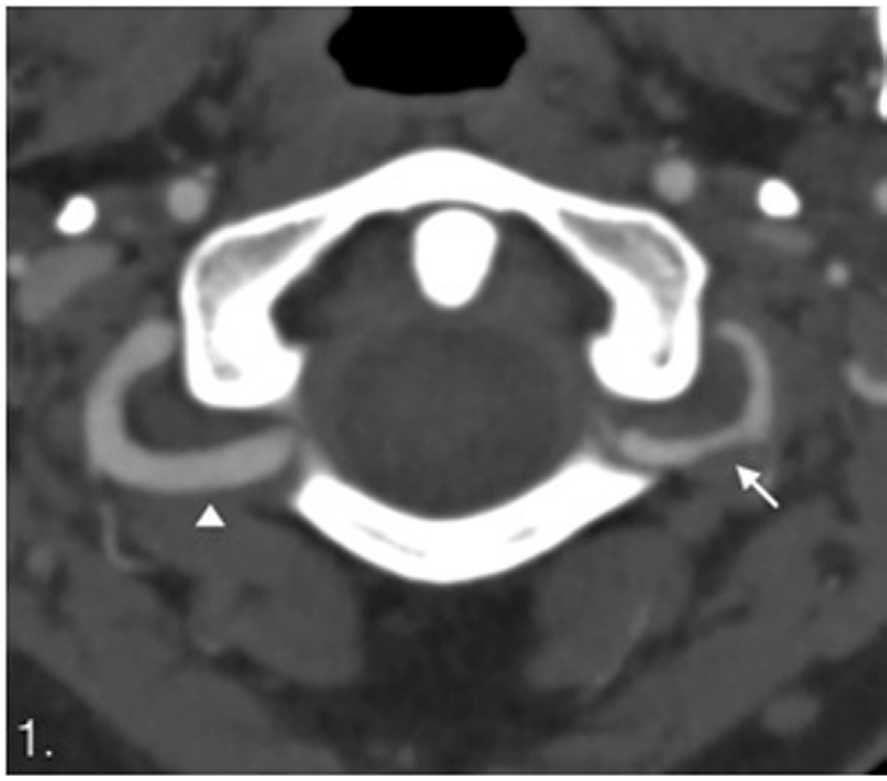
Computed tomography angiography. Dissection of the left vertebral artery with irregular lumen and stenosis (arrow) compare with normal right vertebral artery (arrowhead).
